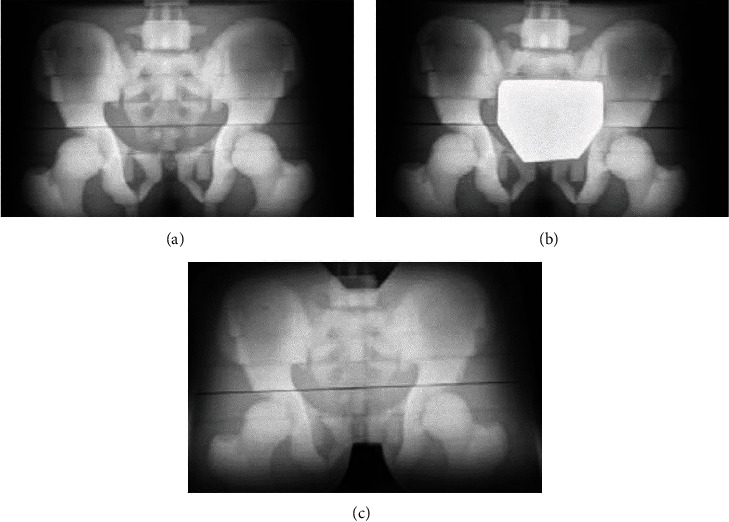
Representative X-ray images from different shielding materials: (a) radiography without any protection, (b) using conventional lead shielding tablets, and (c) using 4-layer bismuth shielding tablets; front and side shielding.
